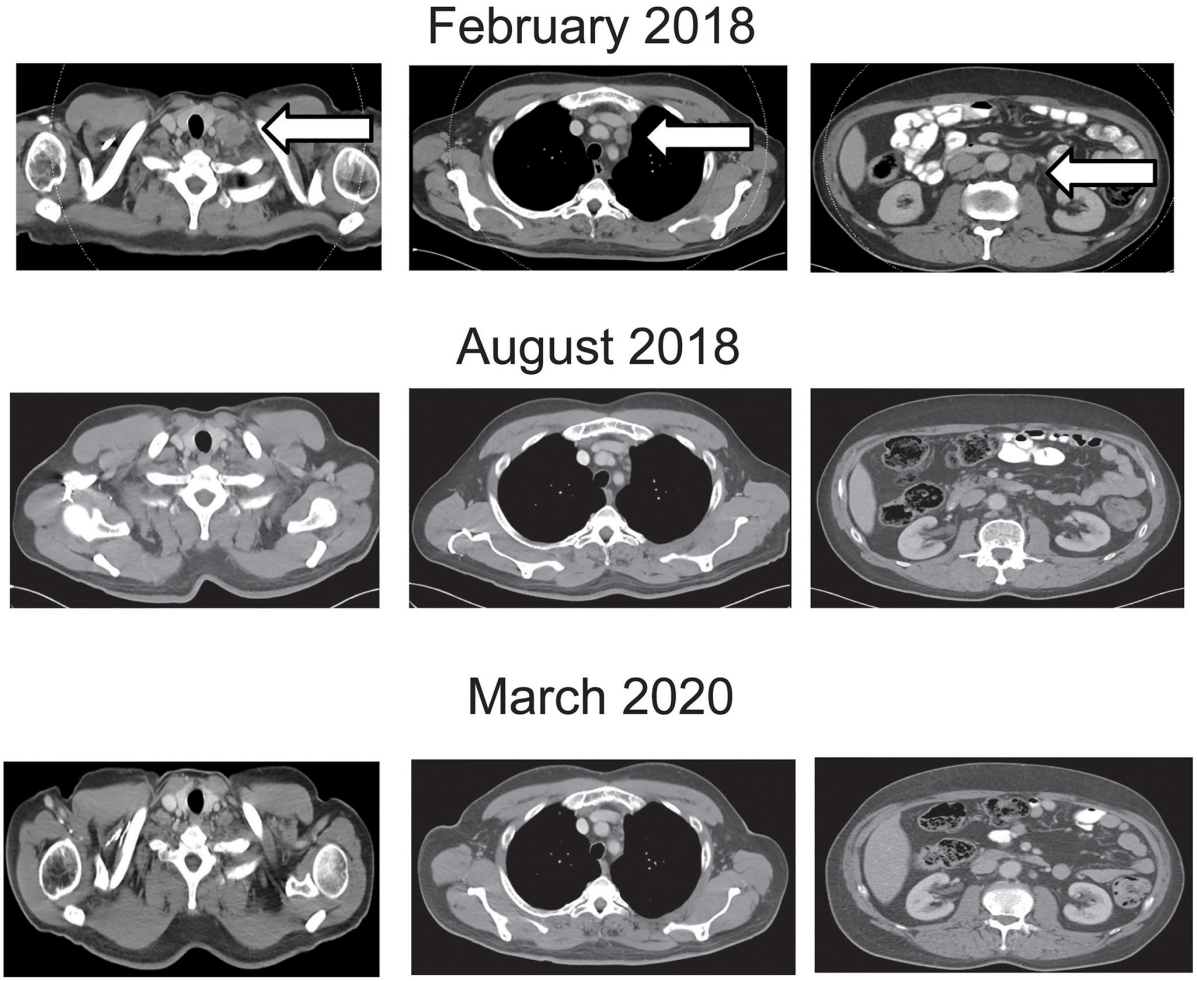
Contrast enhanced computed tomography of the chest and abdomen at beginning, during and at the end of nivolumab treatment. Representative lymph node metastases are shown before, during at end of treatment. Arrows show the metastatic sites before start of treatment.
